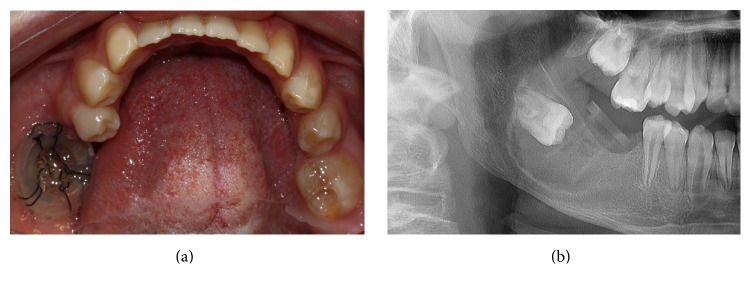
Clinical (a) and radiographic (b) aspects of the compression device following 4 months of installation.
